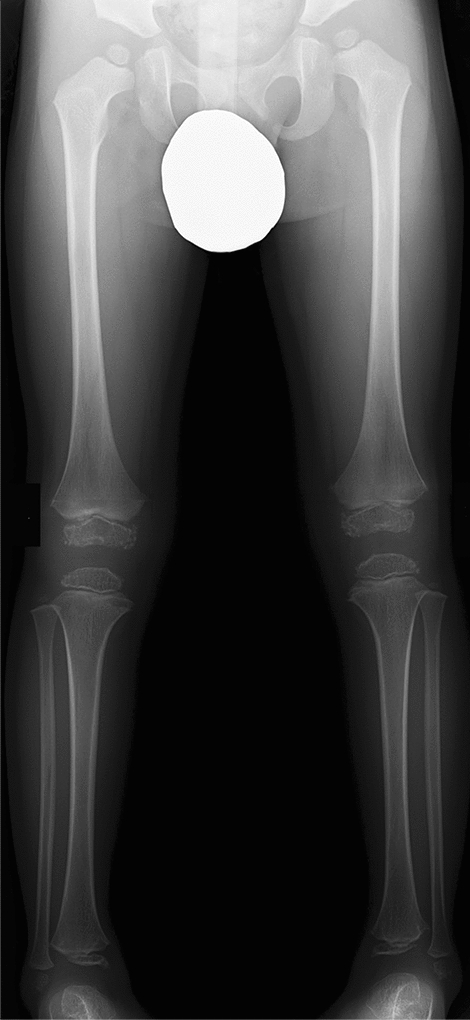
A 5-year-old child with multiple epiphyseal dysplasia, autosomal dominant type. Radiographs show delayed, disorganized epiphyseal ossification including small and round capital femoral epiphyses and irregular epiphyseal ossification of the knee
